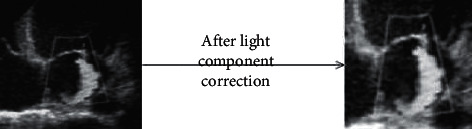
Image correction result (note: the left was the patient's echocardiogram, and the right was the image after the illumination component was corrected).
